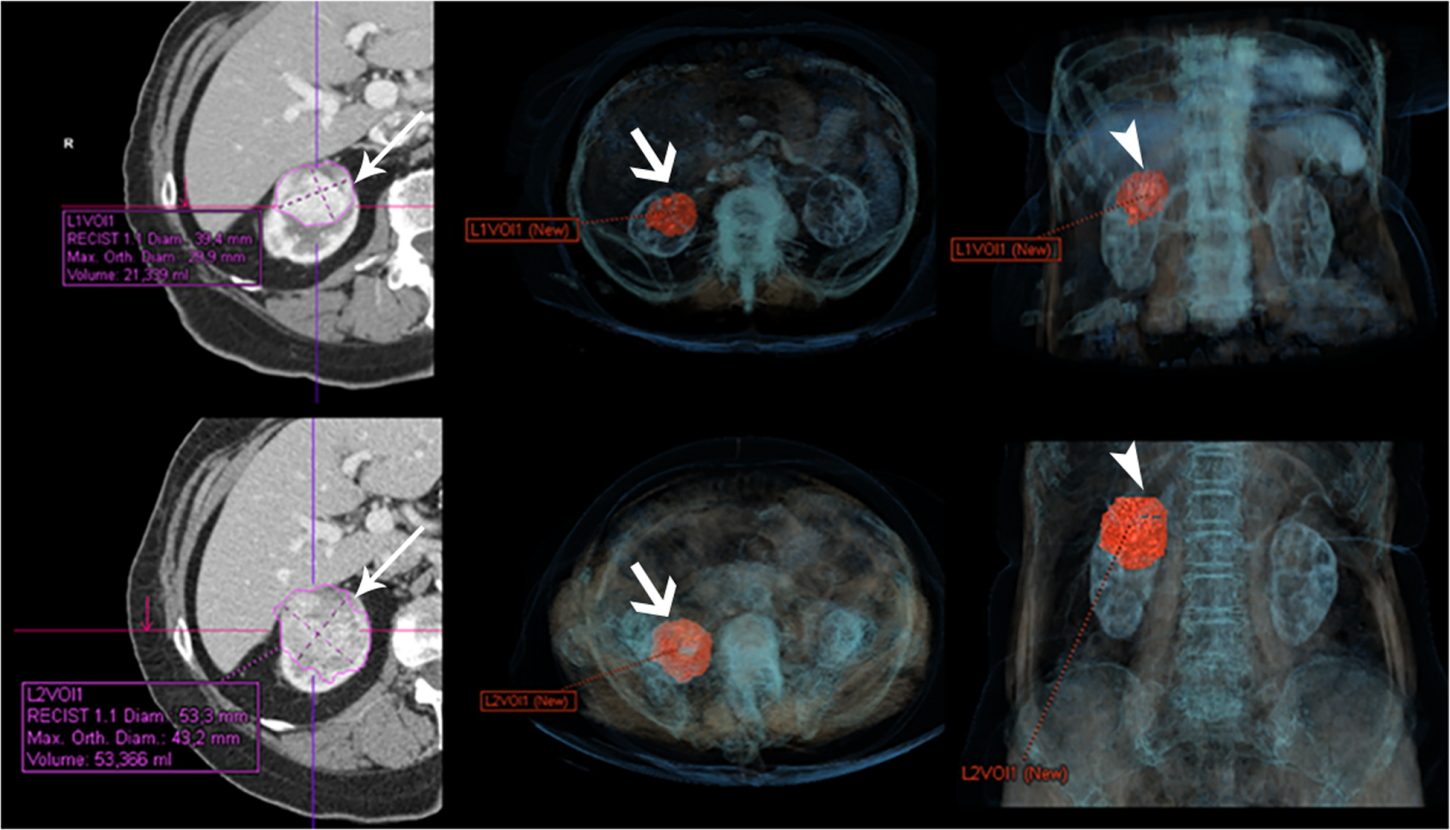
Comparison between linear and volumetric measurements of small renal mass (SRM) growth over a 6-month period. Upper- and bottom-left: axial contrast-enhanced CT showing a renal mass with MTD 3.9 cm at the beginning of AS and 5.3 cm in the next study 6 months later with a linear GR of 1.4 mm between the two studies corresponding to a linear GR of 3.8 cm/year (arrows). Volumetric representation of the renal mass in an axial plane (thick arrows) (upper- and bottom-middle) and coronal plane (arrowheads) (upper- and bottom-right). The software showed an initial volume of 21 cc and a final volume of 53 cc. The increase in volume was 32 cc, indicating that the mass had doubled in volume over a 6-month period representing a rapid growth, although there are no established volume values to define progression
